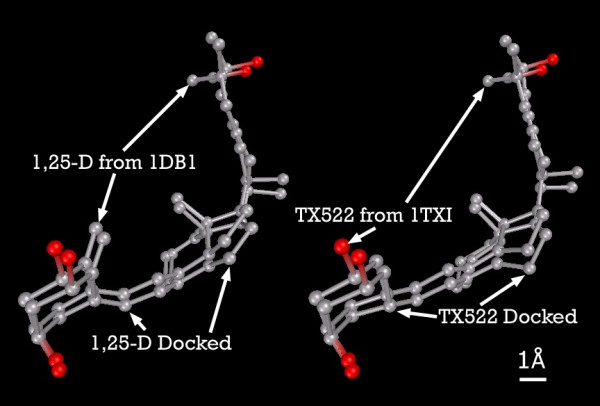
1,25-D and TX522 with superimposed X-ray and VDR-docked configurations. Note: Carbon atoms shown as grey, oxygen as red. Hydrogens not displayed.
